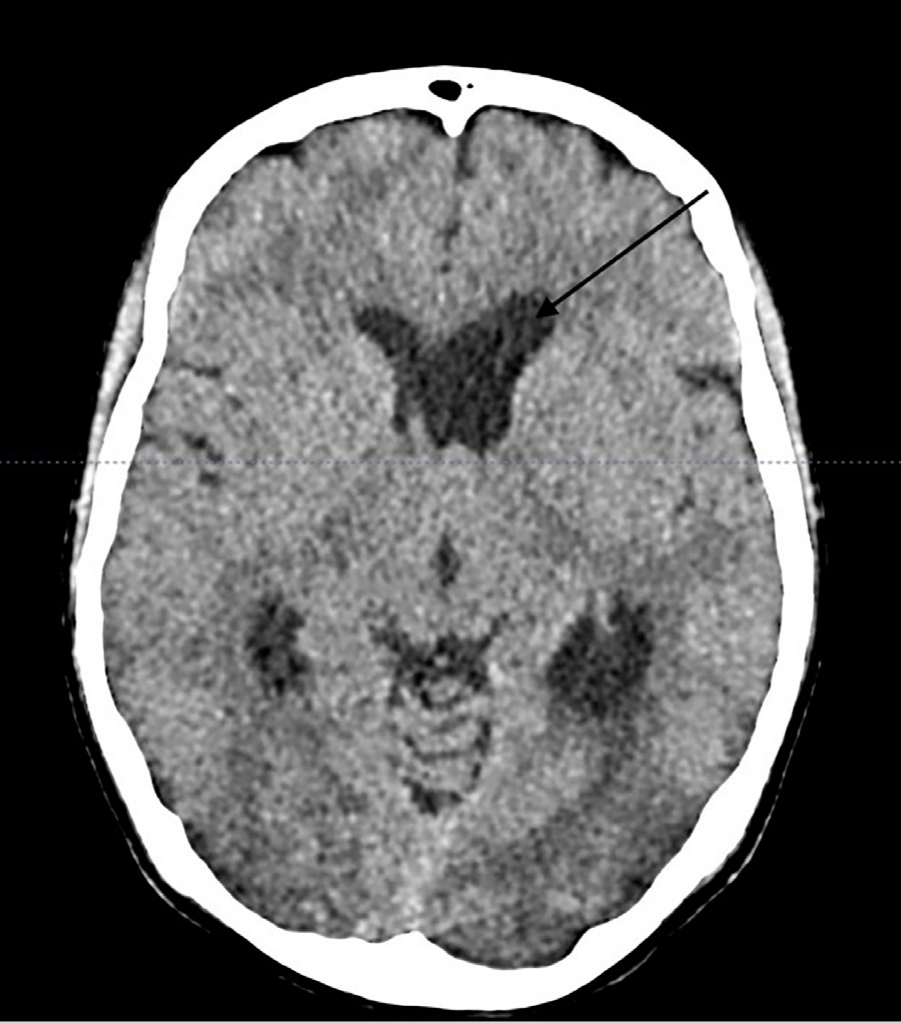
Pre-operative non-contrasted brain CT showing dilatation of the left lateral ventricle, suggesting obstructive unilateral hydrocephalus and suspicion of a small iso-dense lesion at the level of the left foramen of Monro.
